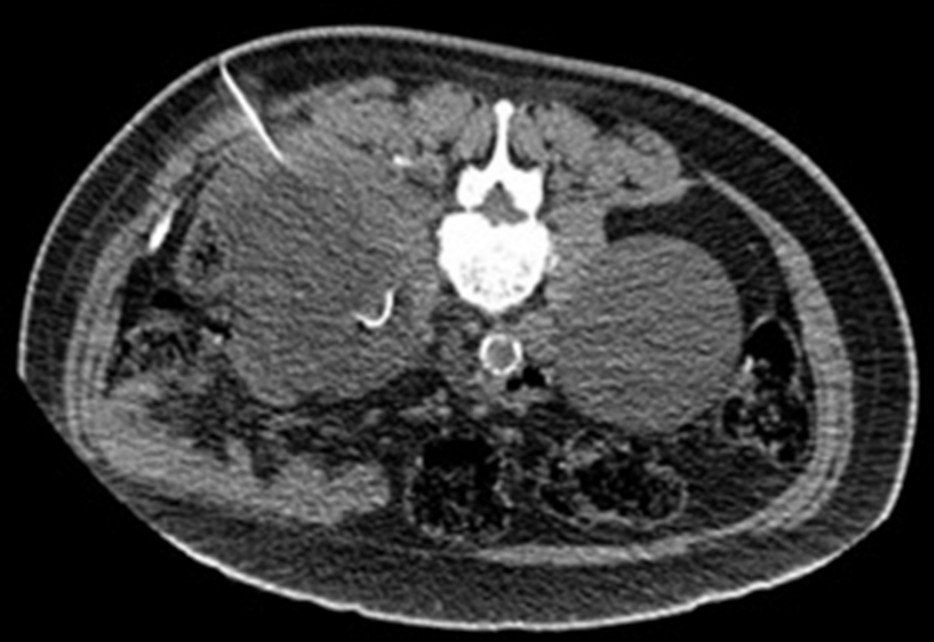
Axial slice of the non-contrast CT abdomen demonstrating the pigtail catheter
